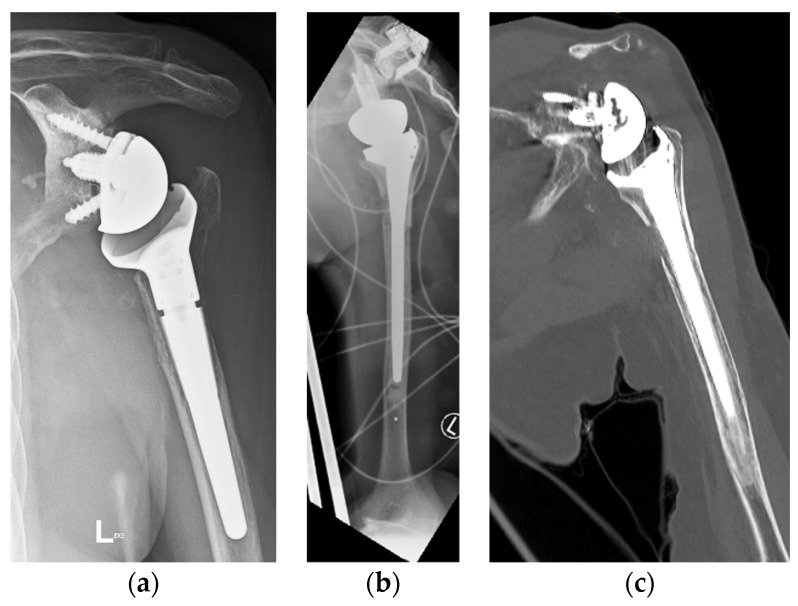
(a) Preoperative anteroposterior (AP) X-ray showing bone loss on the proximal humerus; (b) immediate postoperative AP X-ray showing reconstruction of the proximal humeral defect using an APC; (c) coronal CT scan showing union of the APC with the native humeral shaft.
